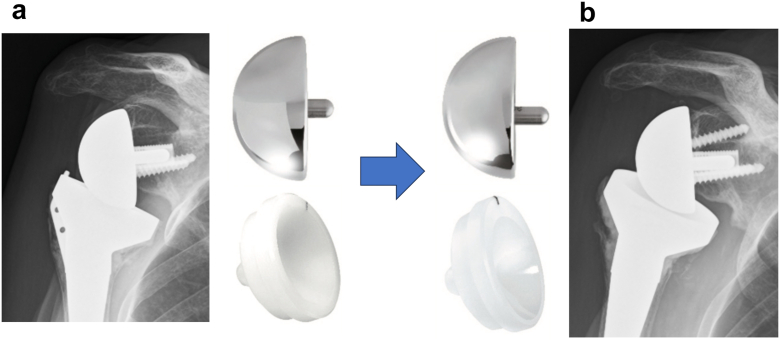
Implant modification to reduce humeral distalization. Pre-operative and post-operative plain radiographs demonstrating changes in the glenosphere position and humeral insert configuration. (a) Pre-operative radiograph showing a standard implant configuration. (b) Post-operative radiograph showing implantation of an eccentric glenosphere in a superior offset position and exchange to a lateralized humeral insert.
